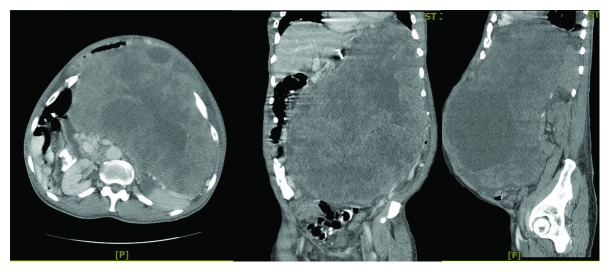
Coronal, transverse, and sagittal views of CT of the abdomen and pelvis showing heterogeneous, partially necrotic mass.
